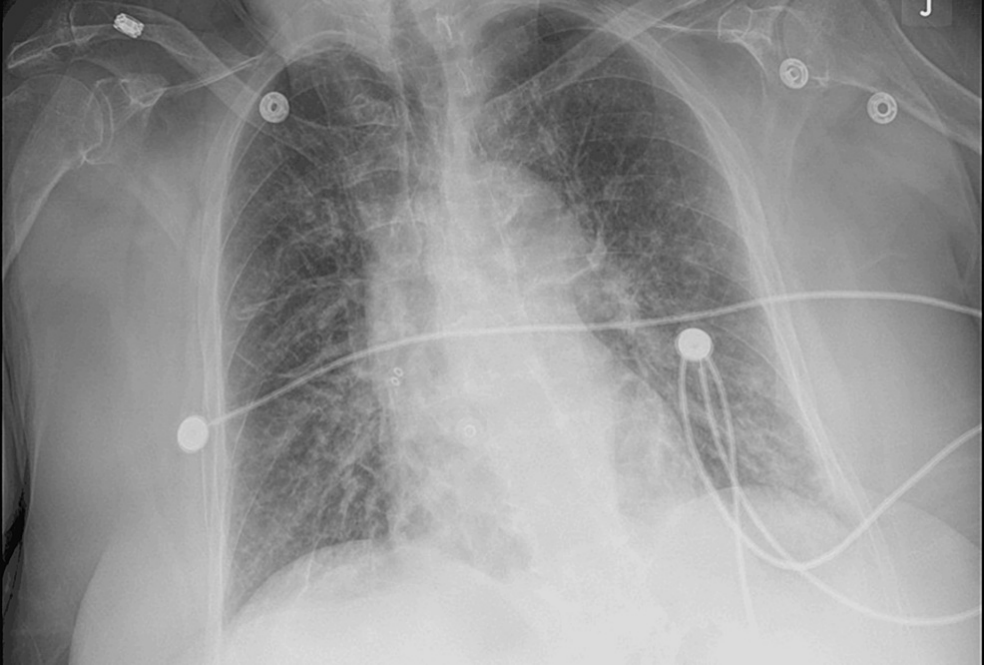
Chest x-ray showing evidence of pulmonary edema.
